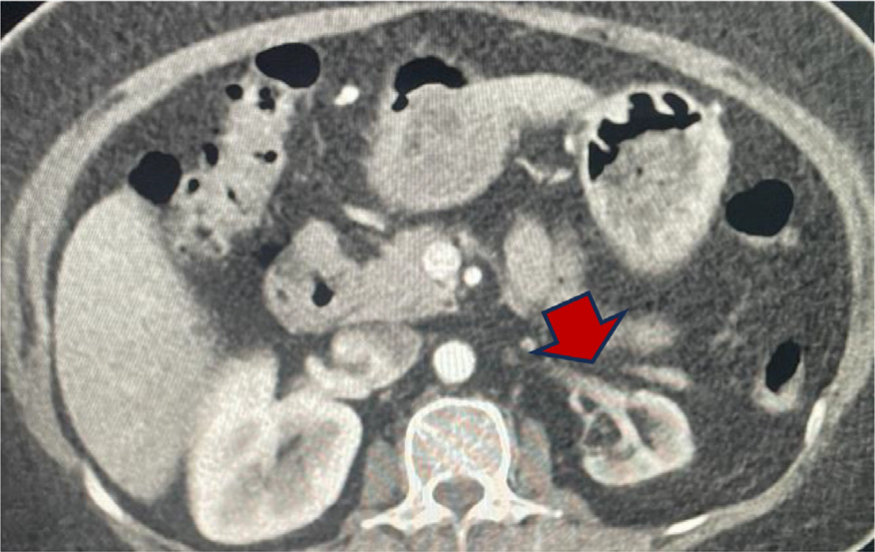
Imaging modality: contrast enhanced computed tomography. Findings: Renal atrophy (red arrow).
